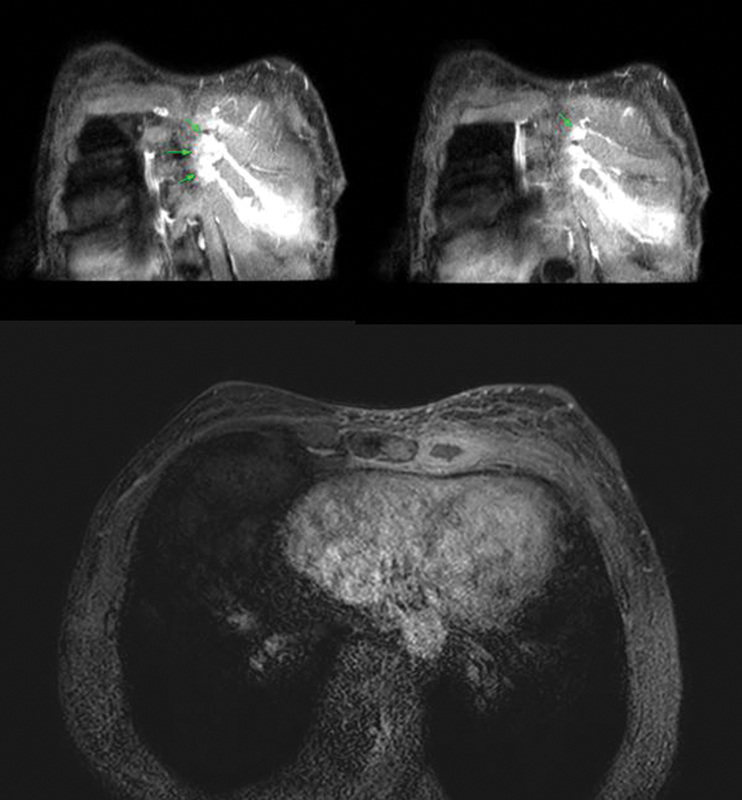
Initial breast magnetic resonance imaging at coronal and axial view has shown increased extent of bone marrow enhancement at left sternal body to fourth costosternal junction and perichondrial enhancement around left costal cartilage, probably due to infection.
